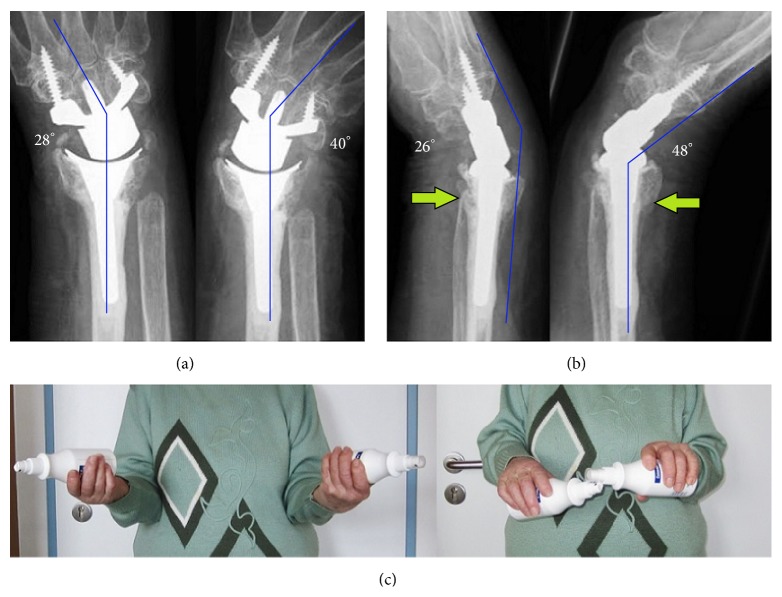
Case report 2 (1-year follow-up): (a) AP radiographs with terminal range of motion: no signs of loosening of either implant and no radioulnar impingement; (b) lateral radiographs with terminal range of motion: no subluxation of distal ulna stump (*arrows*); (c) clinical photo with supination-pronation arc on both sides.
